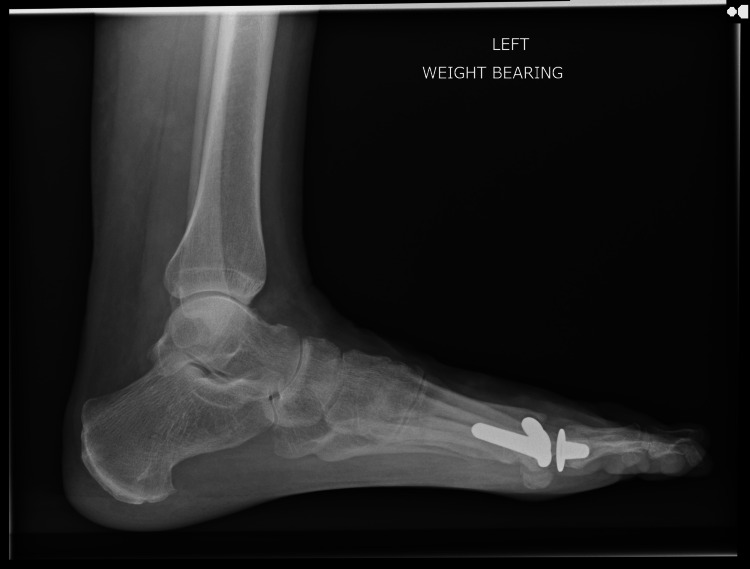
Lateral post-operative X-ray at 36 months following first metatarsophalangeal joint replacement.
